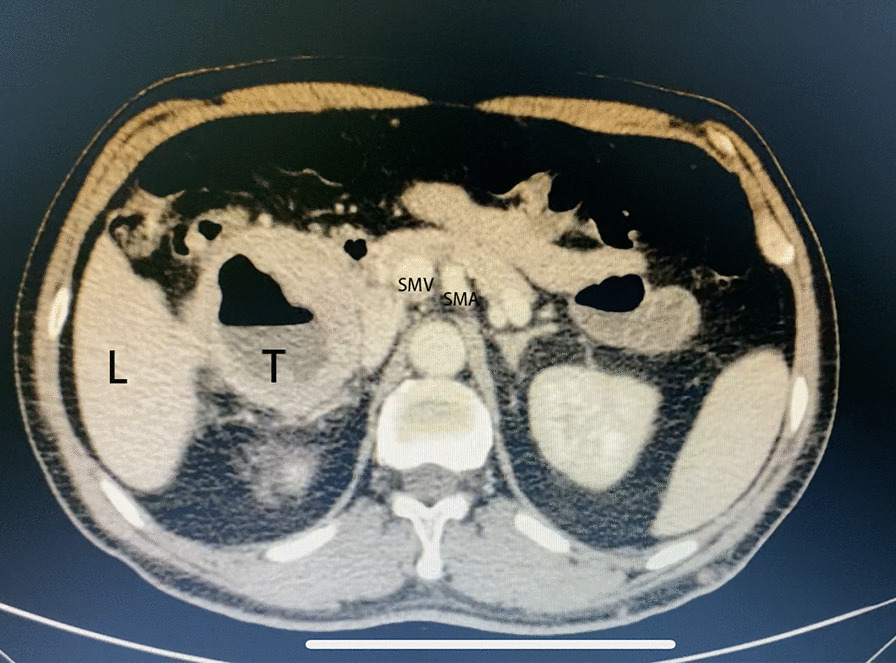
CT image of patient selected for laparoscopic pancreaticoduodenectomy via anterior approach. *L* Liver, *T* Tumor, *SMV* Superior mesenteric vein, *SMA* Superior mesenteric artery
